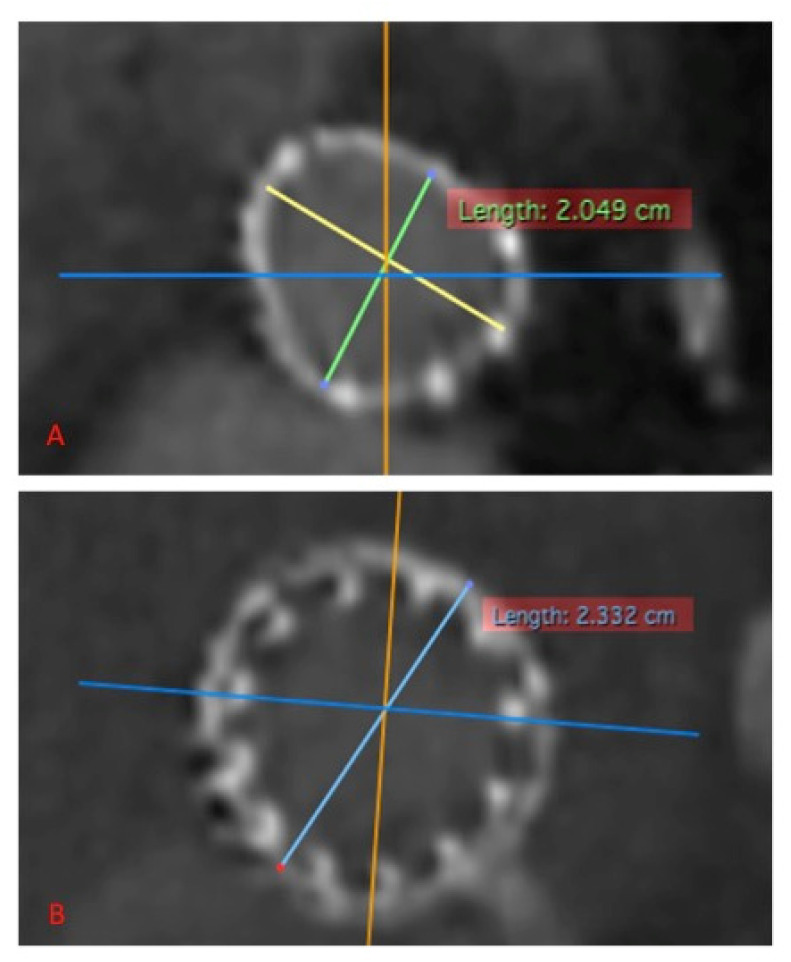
Preprocedural CT scan shows the inflow ring of a degenerated Perceval; a certain level of eccentricity is clearly visible with a sort of rounded triangular shape. (A) Post VIV-TAVIR CT scan of the same prosthesis, we can appreciate a circumferential expansion with minor diameter passing from about 2.05 cm to about 2.3 cm (B).
